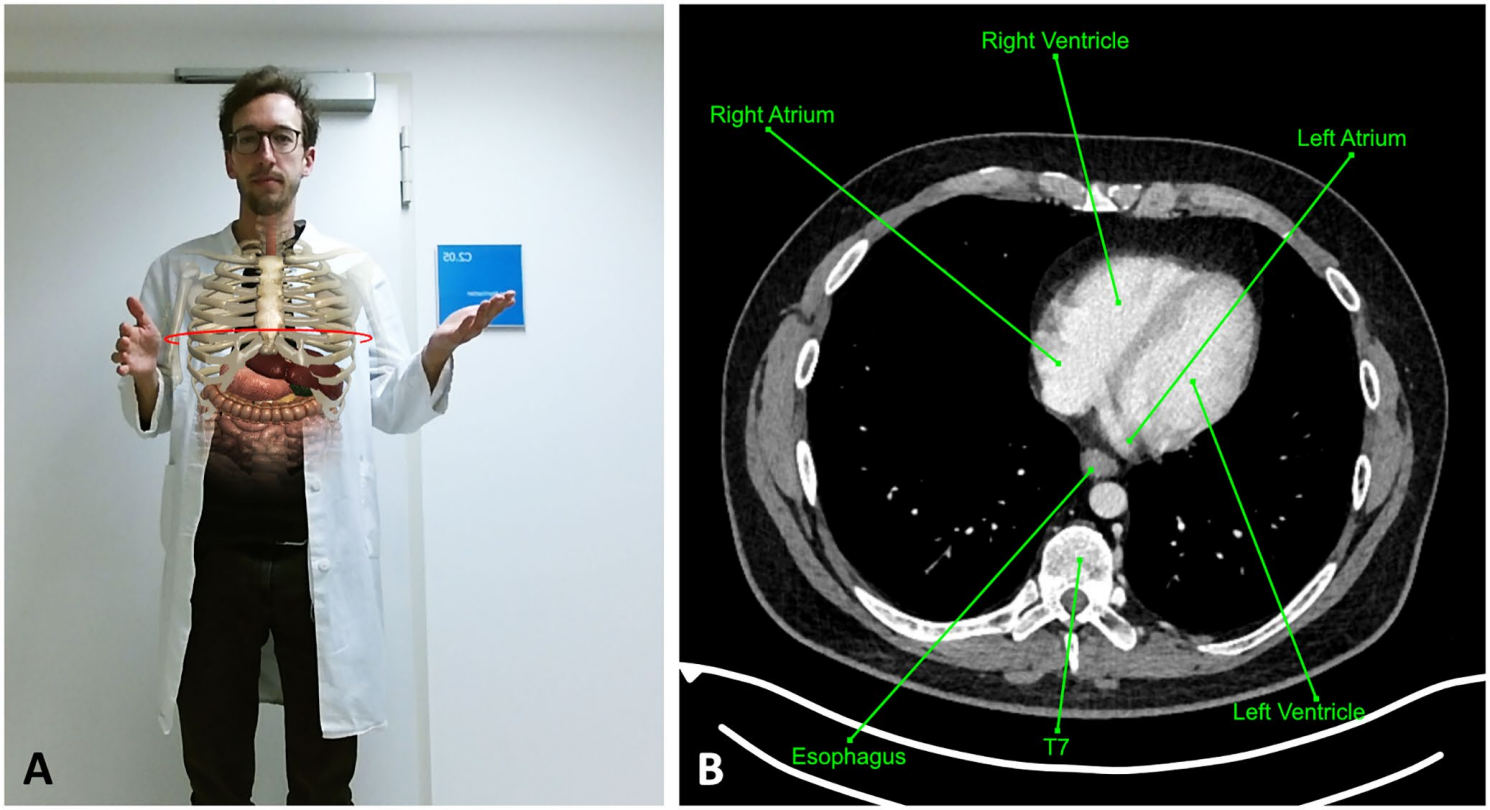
Screenshot of the magic mirror system. A, Augmented Reality (AR) view with virtual anatomy models superimposed on top of the digital mirror image of the user. B, annotated CT section image corresponding to the slice at the height of the virtual red circle in the AR view, controlled via intuitive hand gestures.
